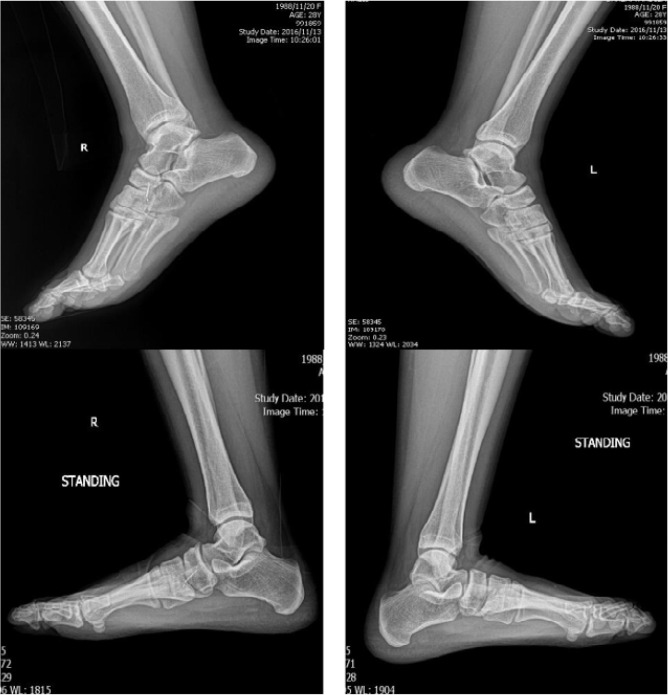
Lateral foot X-ray of both feet in lying (unloaded) and standing (loaded) position.
